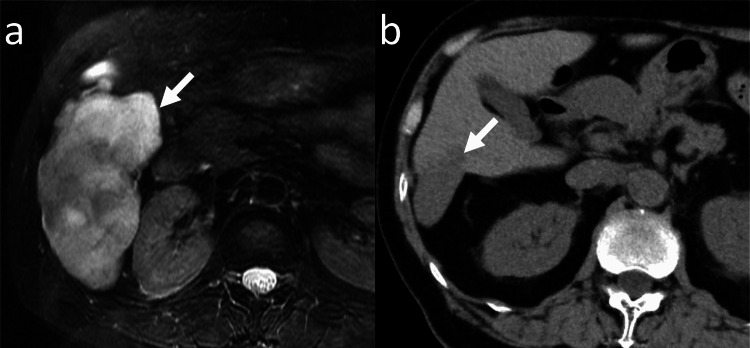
Shrinkage of a hepatic hemangioma in a 57-year-old woman (Case 15 in Table 1) (a) Axial fat-saturated T2-weigheted magnetic resonance imaging (baseline study) of the upper abdomen shows a hepatic hemangioma (arrow) with a diameter of 106 mm in the right lobe of the liver. (b) Axial unenhanced computed tomography (final study) obtained at the 188-month follow-up shows shrinkage of the hepatic hemangioma (arrow). The diameter of the hepatic hemangioma is 46 mm.
